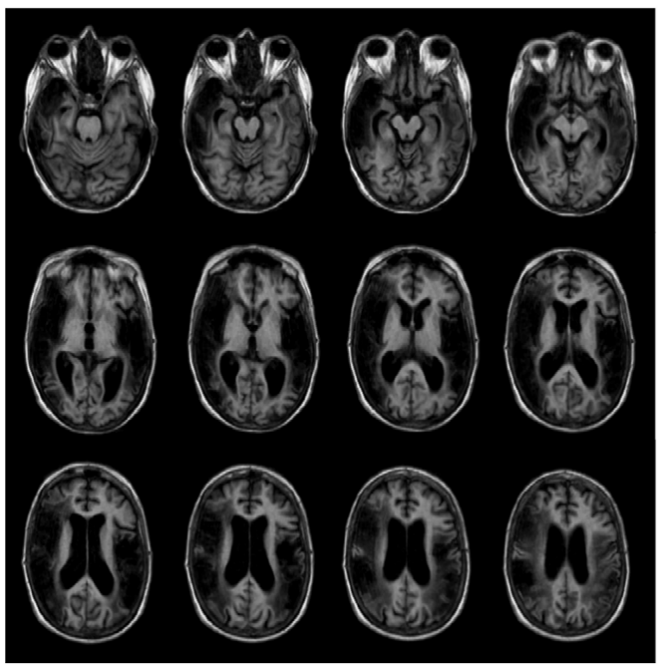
T1-weighted MRI scans of the patient. Consecutive sequences of axial T1-weighted MRI scan of the patient's brain three years after her strokes (right hemisphere is on the left side of the picture). Hypodense lesion massively extends from the bilateral superior temporal gyrus toward the transverse temporal gyrus, the pulvinar, thalamus and insula and the middle temporal gyrus. The right hemisphere was further damaged in the lentiform nucleus, the postcentral gyrus, the inferior frontal gyrus and the inferior parietal lobe.
